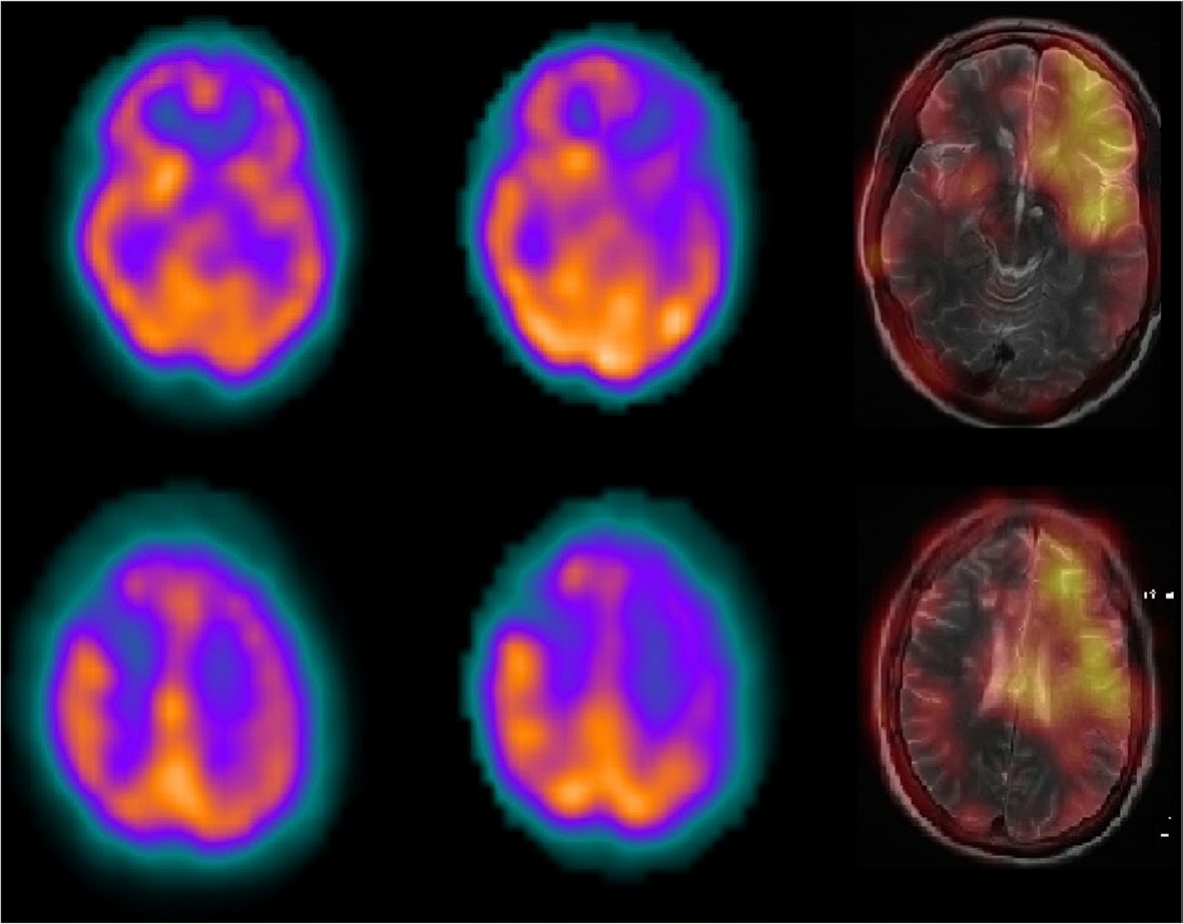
Two axial images of 99mTc-ethyl cysteine dimer cerebral perfusion SPECT/CT at rest (left) with acetazolamide challenge (middle) and a subtracted image of resting perfusion minus acetazolamide challenge with fusion to the T2 weighted MRI sequences (right) demonstrating the area of acetazolamide induced hypoperfusion
